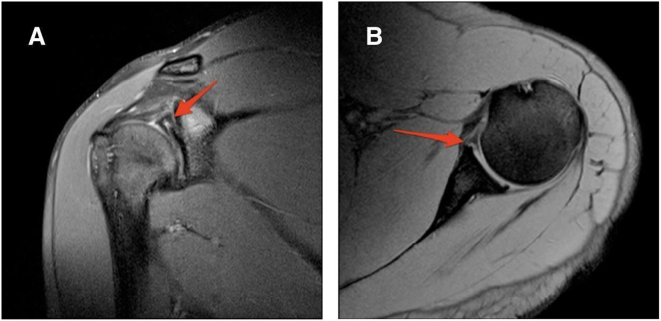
A: Coronal cut of right shoulder MRI showing Bucket-Handle tear of the labrum, Hill-Sach lesion. B: Axial cut of right shoulder MRI showing Bankart lesion.
